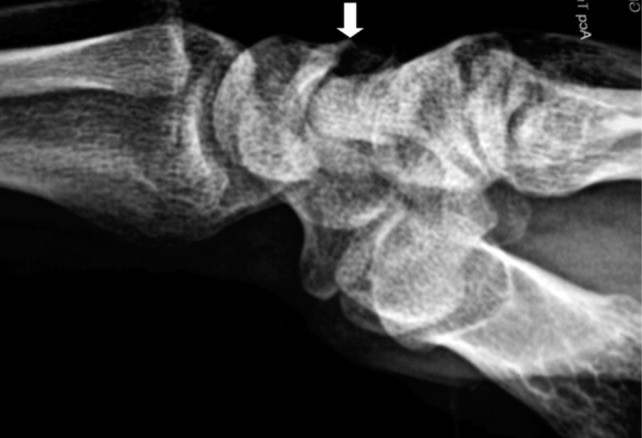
The arrow on the lateral radiograph of the right wrist indicates an osteophyte projected from the dorsal lip of the lunate bone.
